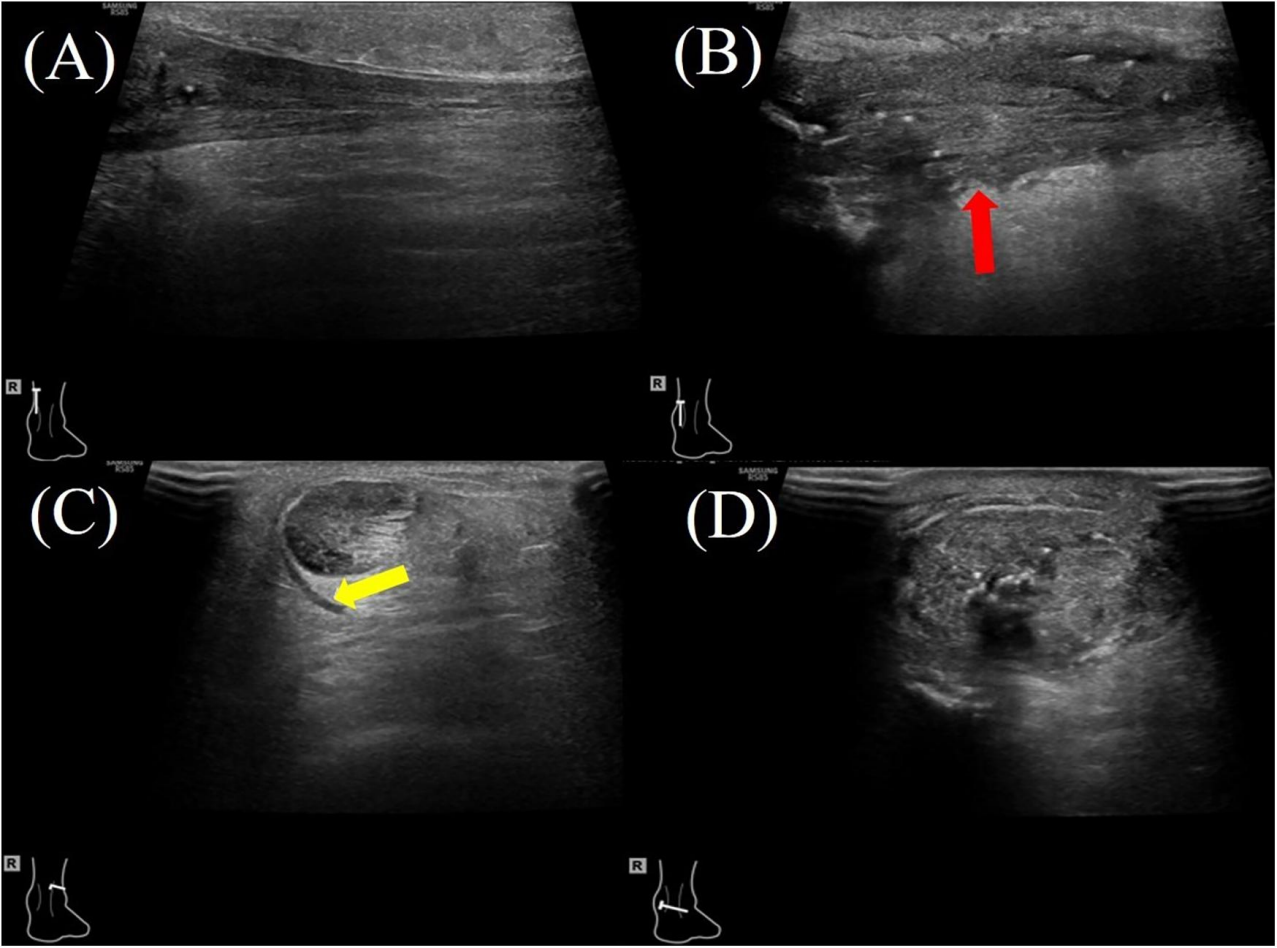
A 47-year-old female patient with right Achilles tendon rupture located 3.3 cm proximal to the calcaneal insertion underwent surgical repair with hAM augmentation. At the 2-month postoperative follow-up, ultrasound evaluation showed: (A) the proximal healthy tendon on the longitudinal plane showing a homogeneous echotexture. (B) the repair site (red arrow) on the longitudinal plane demonstrating mild heterogeneity in echotexture and variable tendon thickness; (C) the proximal healthy tendon on the transverse plane with a small amount of surrounding inflammatory effusion (yellow arrow). (D) the repaired tendon on the transverse plane, demonstrating increased cross-sectional area.
